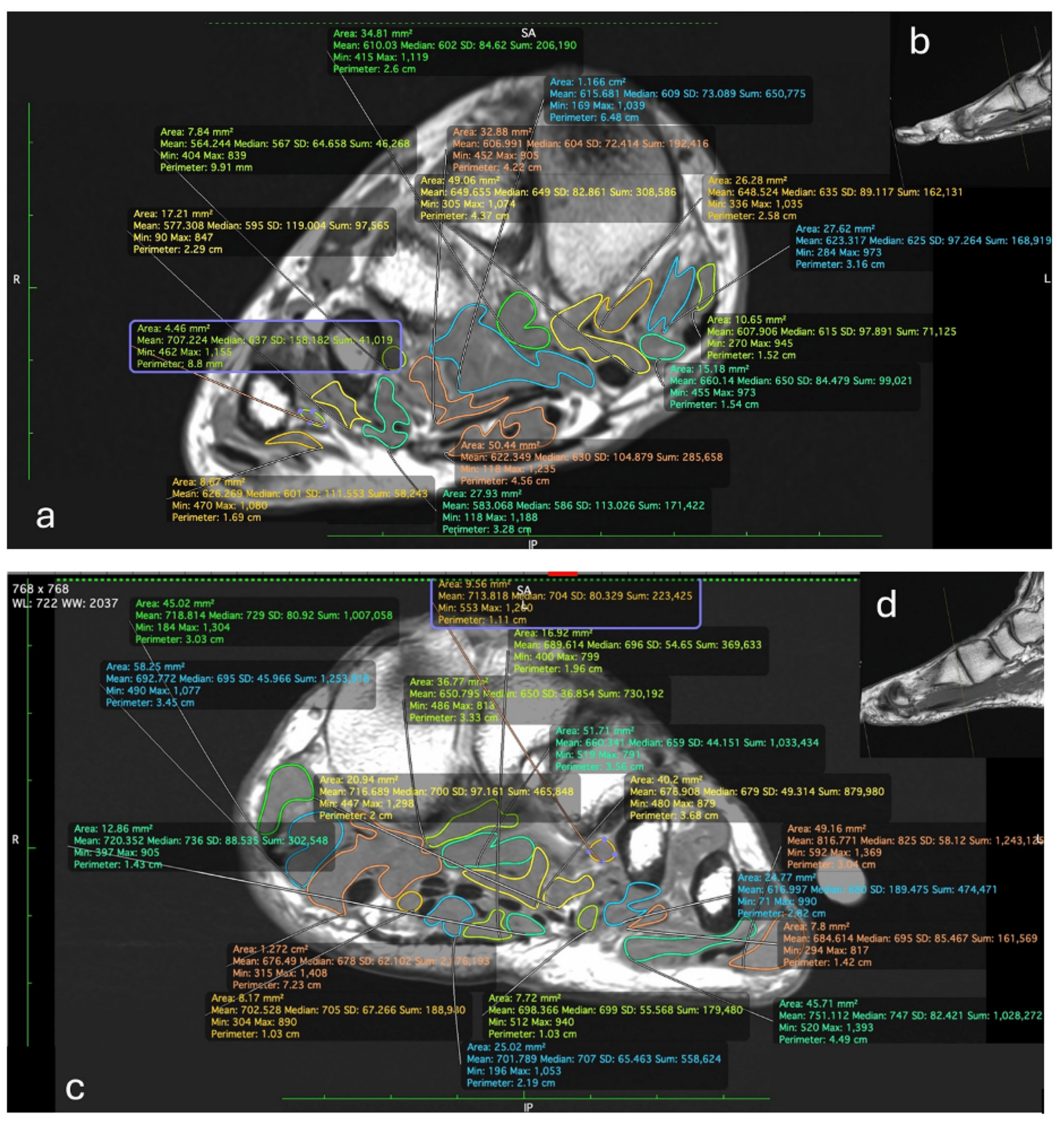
Foot muscle segmentation on coronal magnetic resonance imaging (MRI) of a healthy patient (a) and a patient with a history of falls (c). Measurement level at the first tarsometatarsal joint was confirmed on sagittal views (b and d). Segmented areas were summed to determine the total muscle cross-sectional area.
